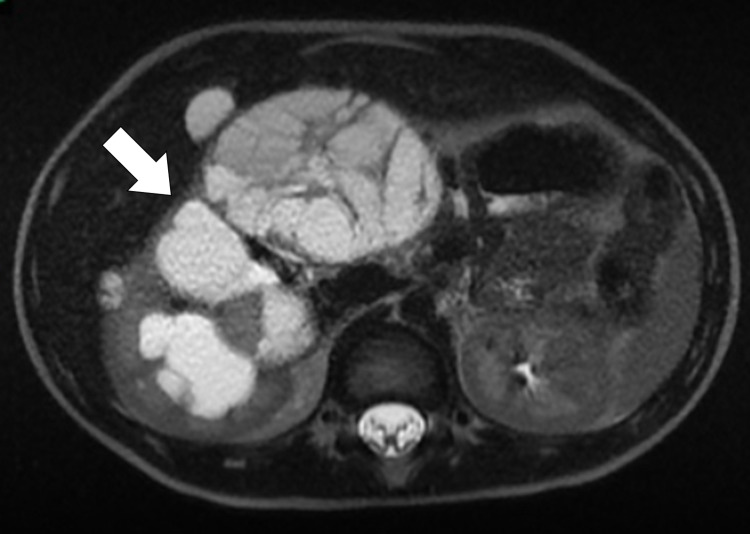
Axial T2 weighted MRI image demonstrates a well-circumscribed, encapsulated mass consisting of multiple cysts with variably enhancing septa soft tissue without vascular or adjacent organ invasion
